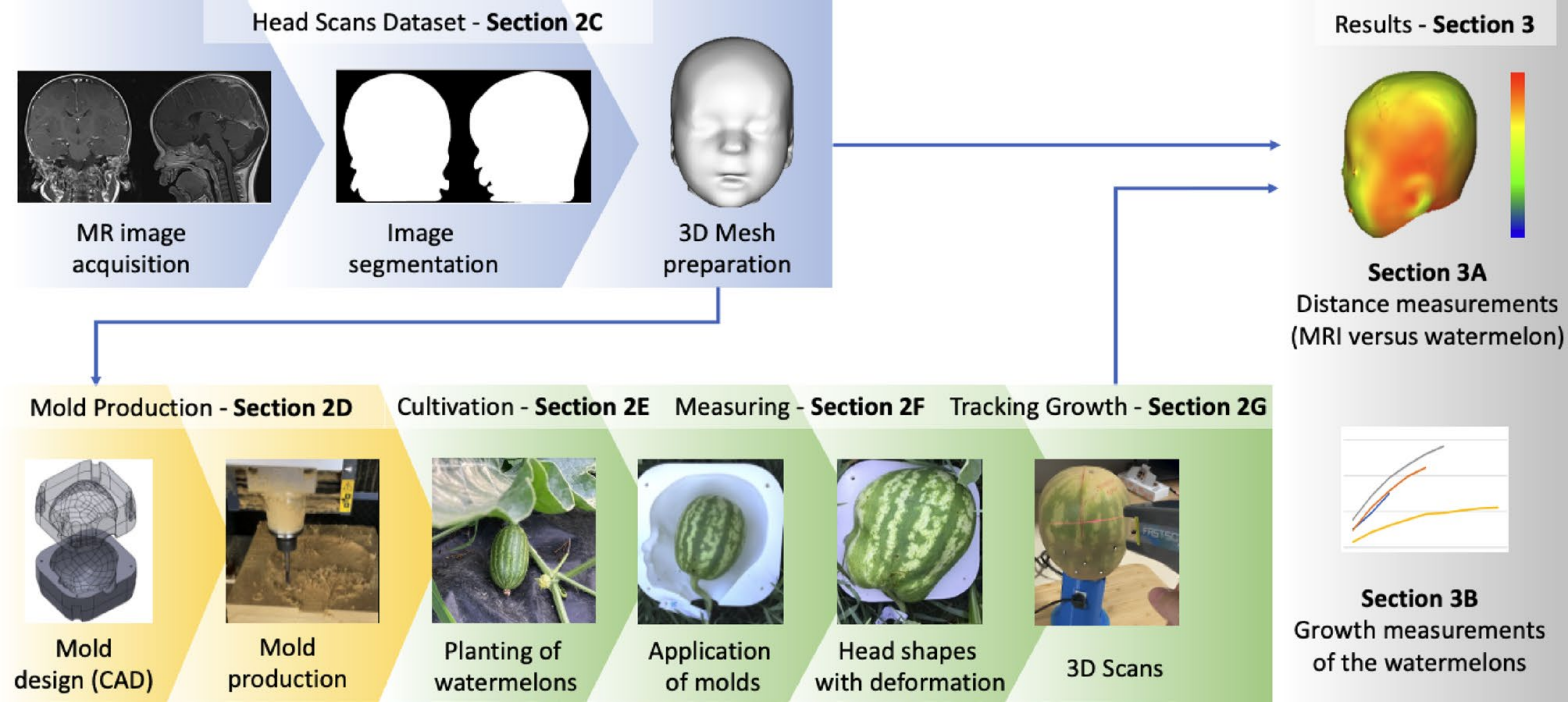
Overview of the method used in this work. MRI images (top left) from the Department of Neonatology and Pediatric Intensive Care Medicine, University Hospital Carl Gustav Carus (Medical Faculty of TU Dresden, Germany). 3D image (top right) generated with Cloud Compare *software* (version 2.12.4) [GPL software] (2021) retrieved from http://www.cloudcompare.org/). 3D model of mold designed in Solidworks version 2020 (*Solidworks*, SolidWorks Corporation, Dassault Systèmes – www.solidworks.com) and photos in bottom row created by the authors.
